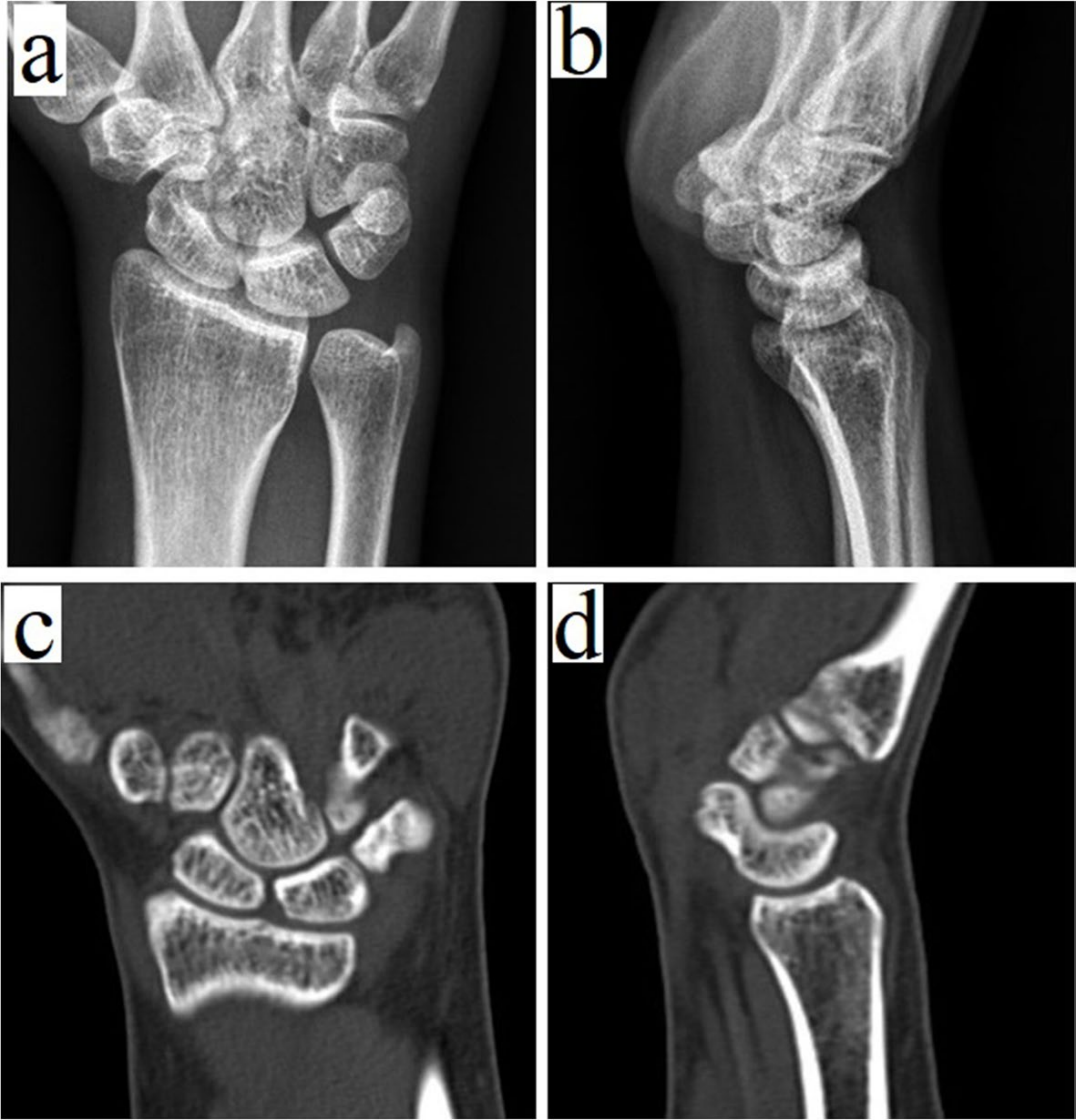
A 29-year-old female. (a, b) Radiographs and (c, d) compued tomography of her left wrist 11 months after the injury event showed no identifiable abnormality of the wrist structure
